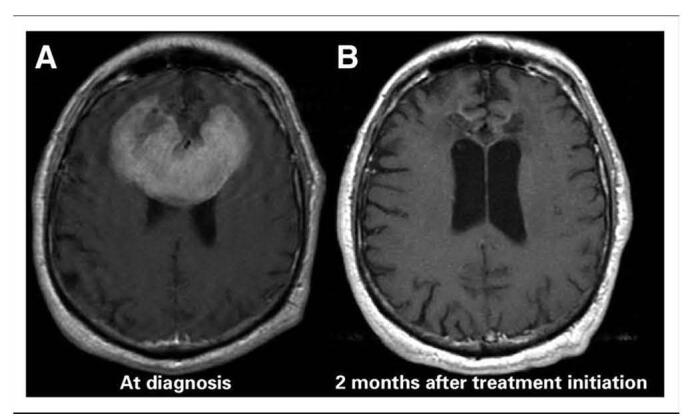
Brain MRI PCNSL. (A) MRI (T1+ gandolinium) displays large, frontal-enhancing brain lesion. (B) Follow-up MRI displays resolution of the large lesion after 2-month treatment with HDMTX. Adapted from Grommes and DeAngelis. (2017). Primary CNS Lymphoma^[21]^.
